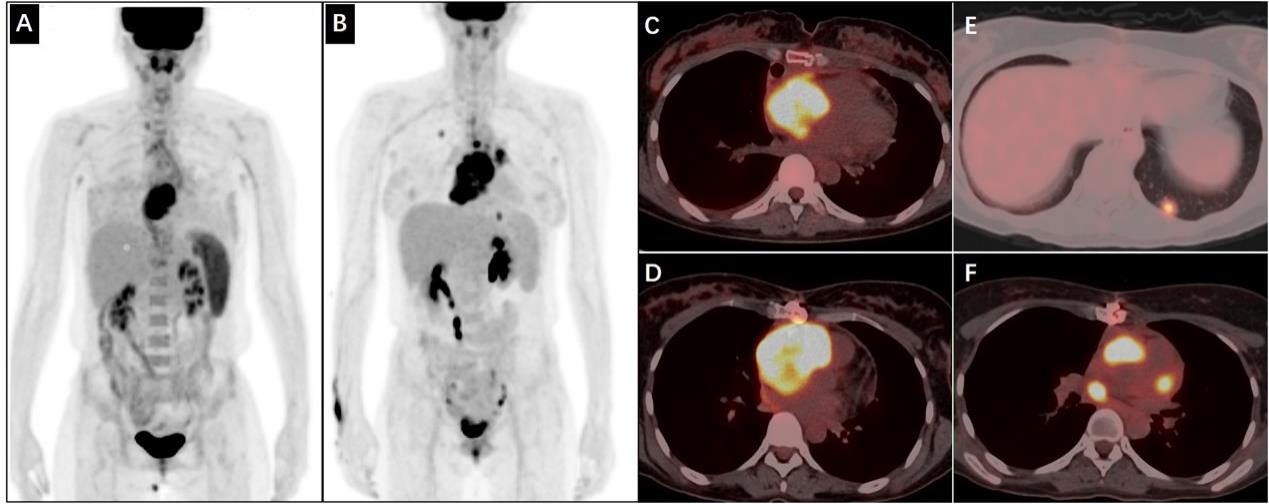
A ^18^F-FDG PET/CT scan. (A, C) Maximum intensity projection (MIP) image and fused PET/CT images revealed intense ^18^F-FDG uptake in the right side of the heart. The maximum standardized uptake value was 10.9. (B, D) MIP image and fused PET/CT images repeated after three months showed the increased ^18^F-FDG uptake had become more extensive, compared with A and C. In addition, multifocal ^18^F-FDG uptake was demonstrated in both lungs and mediastinum. (E, F) Multiple nodules with clearly increased ^18^F-FDG activity in the lungs and mediastinum were newly visualized.
